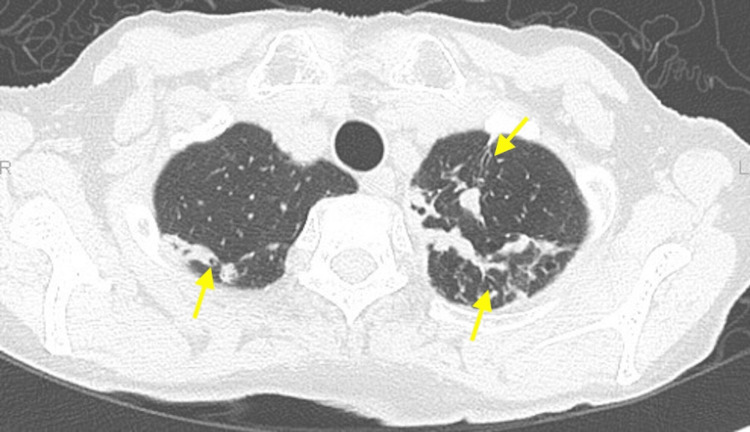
Chest computed tomography scan Chest computed tomography scan on admission showing dilated bronchioles surrounded by granular, chordate, and plaque shadows (yellow arrows) in the bilateral lung apexes.
